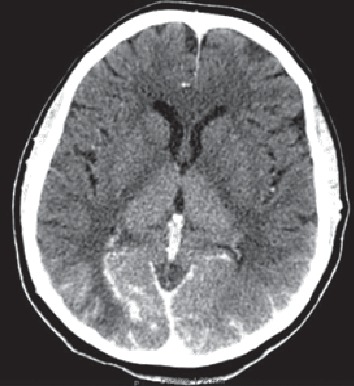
CT (axial) obtained one hour after DSA shows bilateral symmetrical contrast enhancement in parieto-occipital cortex and subarachnoid spaces, as well as in thalami.
